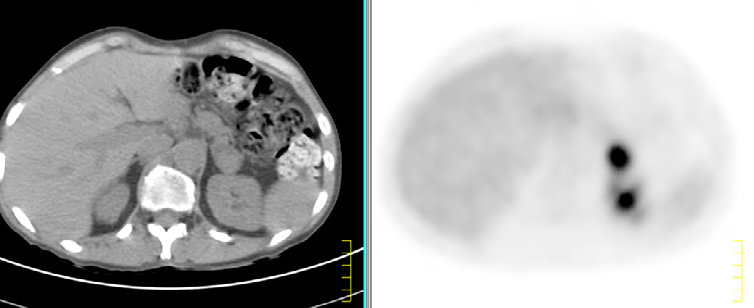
A). CT scan image and B). 18FDG-PET scan.18FDG-PET scan with CT anatomical image fusion (axial view) shows an intense abnormal FDG uptake within the adrenal gland suggestive of metastasis or possibly a primary tumour.
